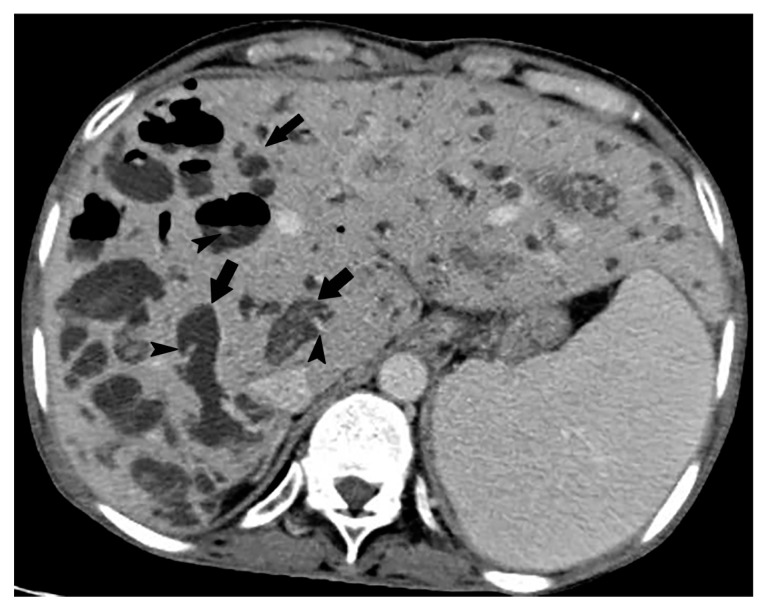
Caroli’s disease in a 58-year-old man. In the equilibrium phase on contrast-enhanced CT, multiple hypodense cystic or tube-like structures (arrows) are observed throughout the liver. Contrast-enhanced portal venous branches (arrowheads) are observed in the center of the hypodense structure, which is called the “central dot sign”, a characteristic finding of Caroli’s disease.
